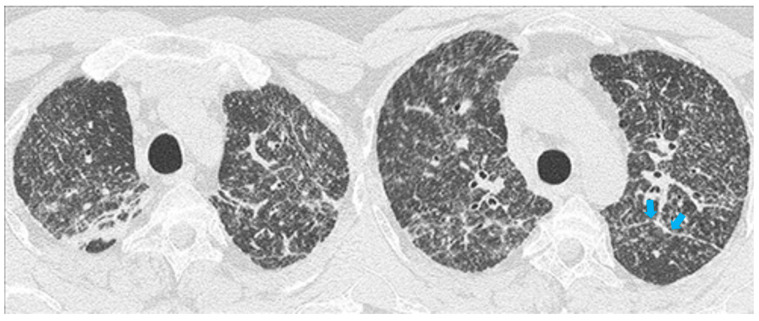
Axial HRCT scans show an advanced-stage sarcoidosis characterized by lung architectural distortion and traction bronchiectasis. Blue arrows show a left fissure displacement.
